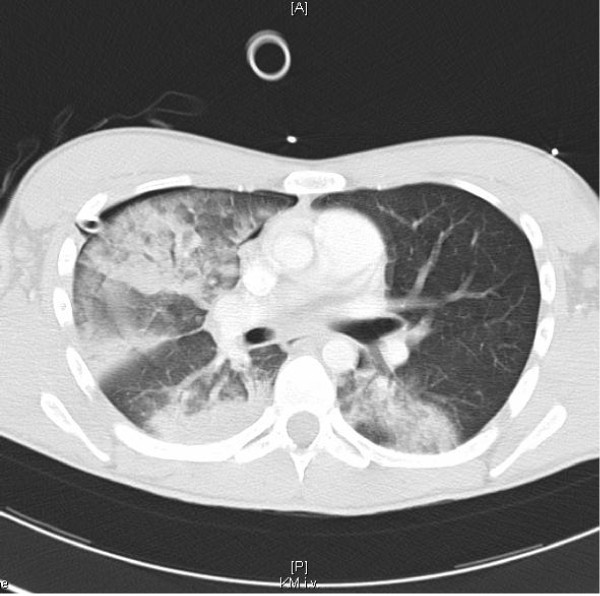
CT findings of the lung edema. A bilateral lung edema can be seen in the CT of the chest.
